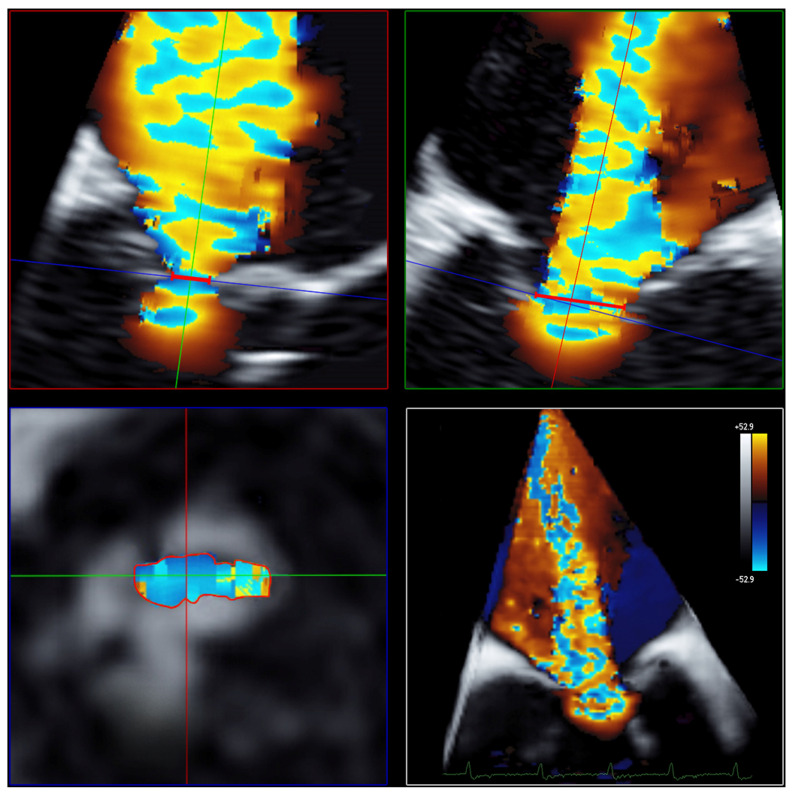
Determination of 3D VCA using multiplanar reconstruction in the 3D color Doppler dataset. Echocardiographic images with 3 slice planes and 3D reconstruction (lower right) of the 3D color Doppler dataset (mid-systolic). Planimetric fitting of the 2 upper planes along the course of the color Doppler jet; third plane (lower left) adjustment orthogonal to planes along the jet and finding the minimal cross-section of the regurgitation jet using translation and tilting of the third plane; manual measurement of the minimal cross-sectional plane using outlining. Abbreviations: 3D = three-dimensional; VCA = vena contracta area.
